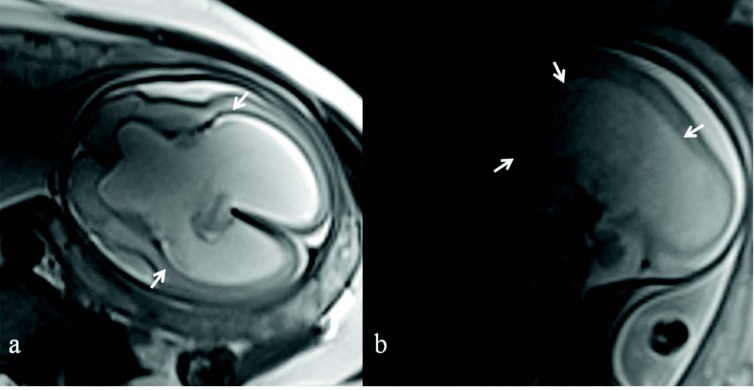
Axial (a) and sagittal (b) T2 HASTE fetal MR images show hydrocephalic dilatation of the lateral ventricles.
